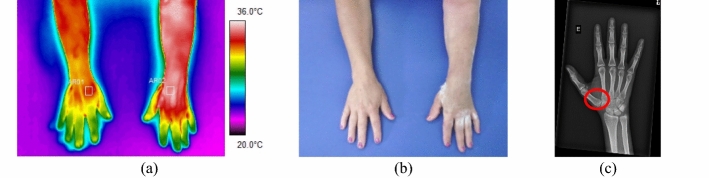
a Shows the thermal image of an acute fracture of the left hand (scaphoid bone) and contralateral limb, with the delimitation of (ROI) defined for comparative analysis; b visible image of the evaluated limb; c shows the radiographic image of the bone trauma
